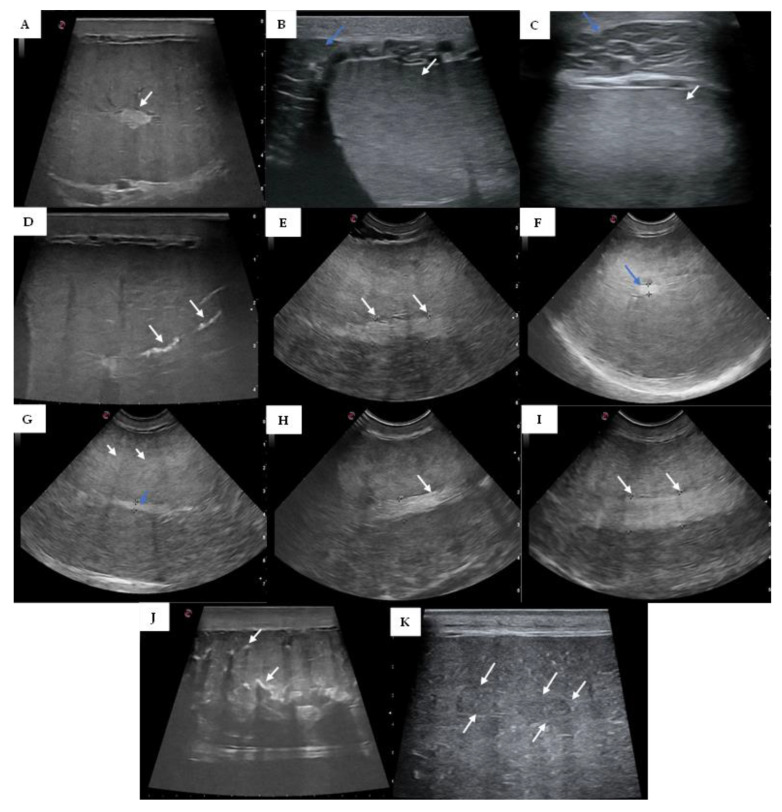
Representative ultrasound images for bulls with homogeneous testicular parenchyma (A–C): (A) medium echogenicity with the mediastinum testis as a central or slightly eccentric hyperechoic focus (white arrow); (B) homogenous testicular parenchyma (white arrow) and head of the epididymis (blue arrow); (C) homogenous testicular parenchyma (white arrow) and body of the epididymis (blue arrow); and bulls with heterogeneous testicular parenchyma (D–K): (D) linear hyperechoic, non-shadowing foci (white arrows) filling the rete tubules indicative of spermiostasis; (E) slight heterogeneous testicular parenchyma and distension of mediastinum testis (8.7 and 8.8 mm left and white arrow respectively); (F) slight heterogeneous testicular parenchyma and mediastinum testis of 4.2 mm (blue arrow); (G) slight heterogeneous testicular parenchyma with small hyperechoic foci (white arrows) and mediastinum testis of 3.6 mm (blue arrow); (H) heterogeneous testicular parenchyma, distension, and thickening of mediastinum testis (6.8 mm, white arrows); (I) bilateral testicular hypotropia and severe mediastinum distension (10.4 and 10.8 mm left and white arrow respectively); (J) coarse echotexture with multiple hyperechoic foci (white arrows) with distal acoustic enhancement scattered throughout the parenchyma; (K) inhomogeneous echotexture with hypoechoic areas scattered throughout the parenchyma.
